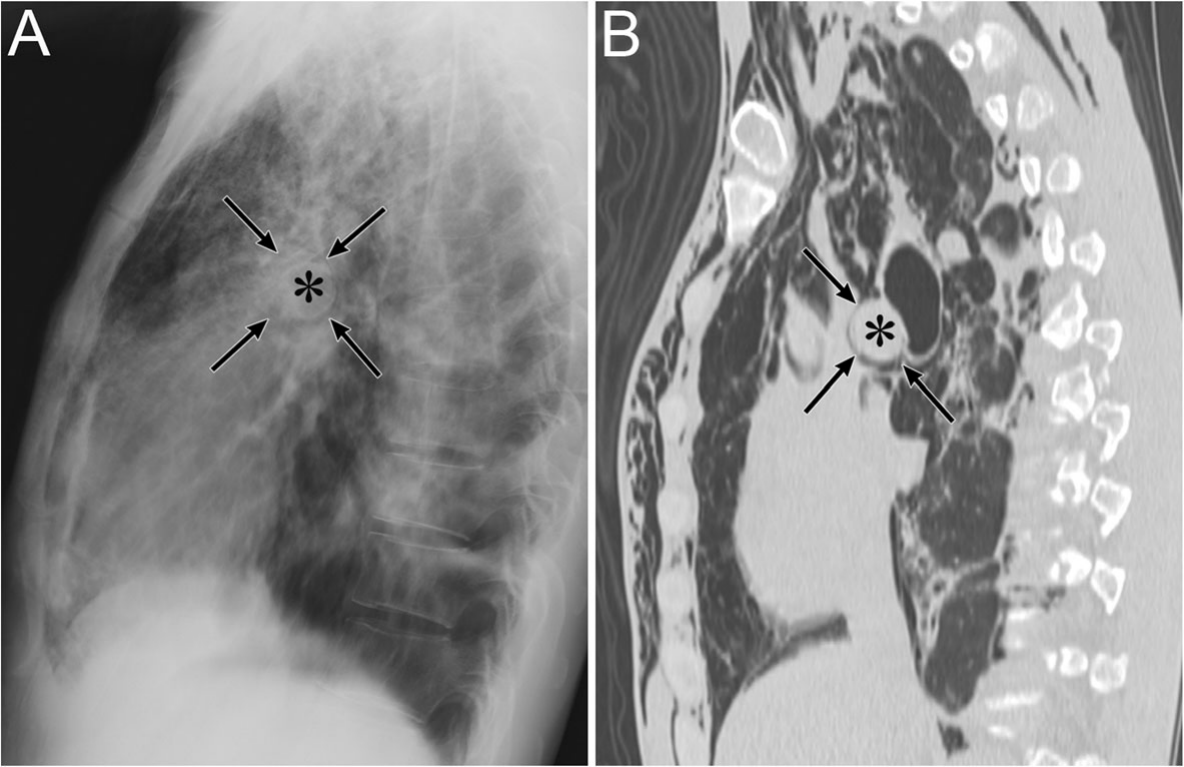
Pneumomediastinum of a 43-year-old man with acute myelogenous leukemia. a Erect lateral radiograph shows the “ring around the artery” sign. b A sagittal CT image also shows a lucent ring (arrows) surrounding the right pulmonary artery (asterisk), a feature called the “ring around the artery” sign
